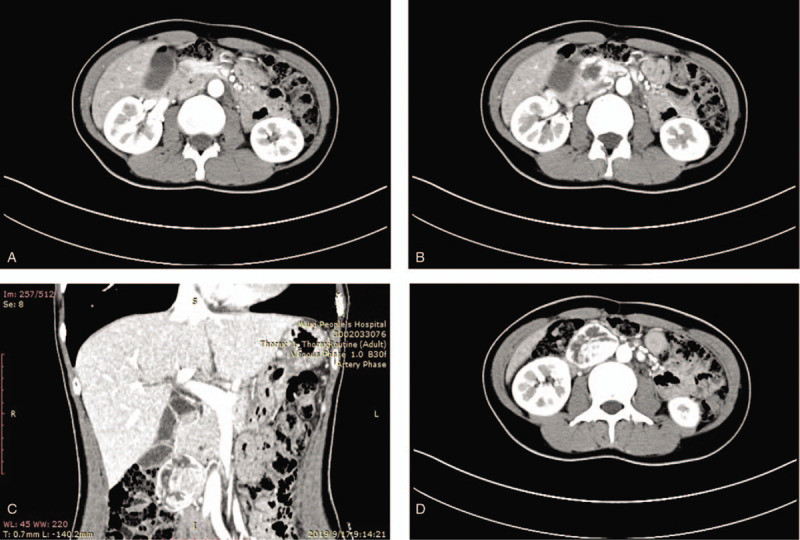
Abdominal computed tomography performed 3 days after admission of the 43-year-old female patient. (A, B, and C) Space-occupying mass lesion below the head of pancreas. (D) The mass had rich blood supply.
